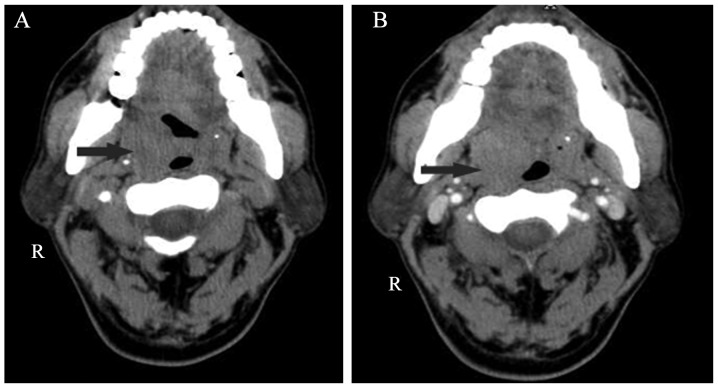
Computed tomography scans revealing a homogenously enlarged 4.6×2.5×2.5-cm right tonsil, which was well-circumscribed. (A) The initial enhancement value was 53 HU, and (B) slight continuing heterogeneous enhancement was evident following injection of contrast medium. R, right.
